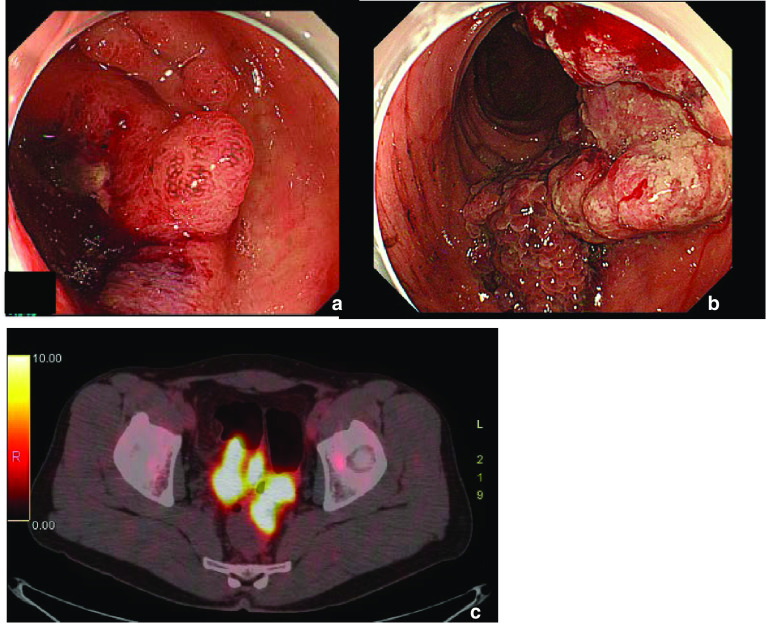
Colonoscopy revealed a circular type 3 lesion in the sigmoid colon (a) and a semicircular type 2 lesion in the rectum (b). A positron emission tomography (PET)-CT scan showed fluorodeoxyglucose (FDG) accumulation in the sigmoid colon lesion, rectal lesion, and swollen lymph nodes (c)
